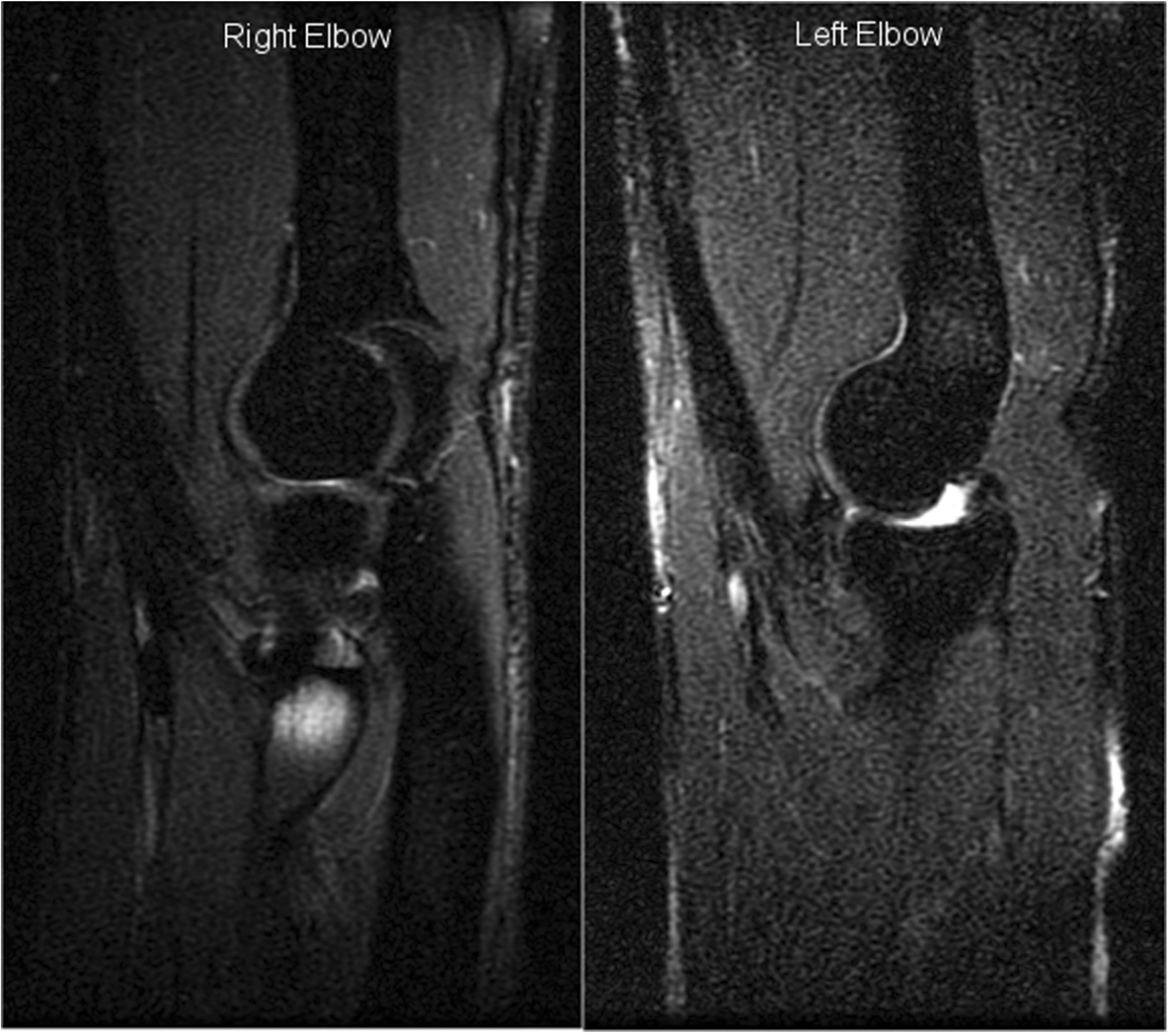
Postoperative MRI at 14 month follow-up demonstrate well proportioned and tight grafts bilaterally. Right elbow is semtendinosus graft and left elbow is quadriceps graft.
